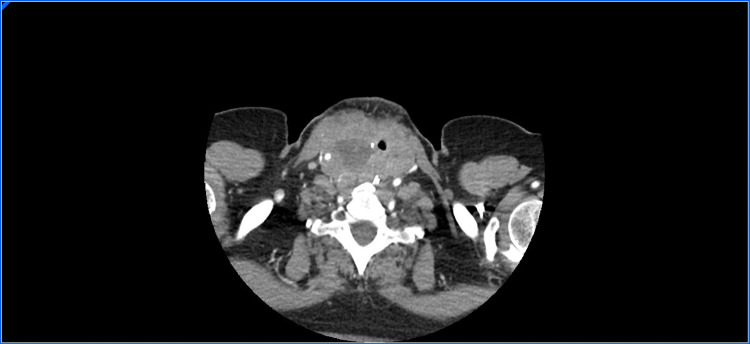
CT neck with contrast scan showing large thyroid lesion with significant tracheal compression, resulting in a minimal lumen size of 6 mm, without direct tracheal invasion.
